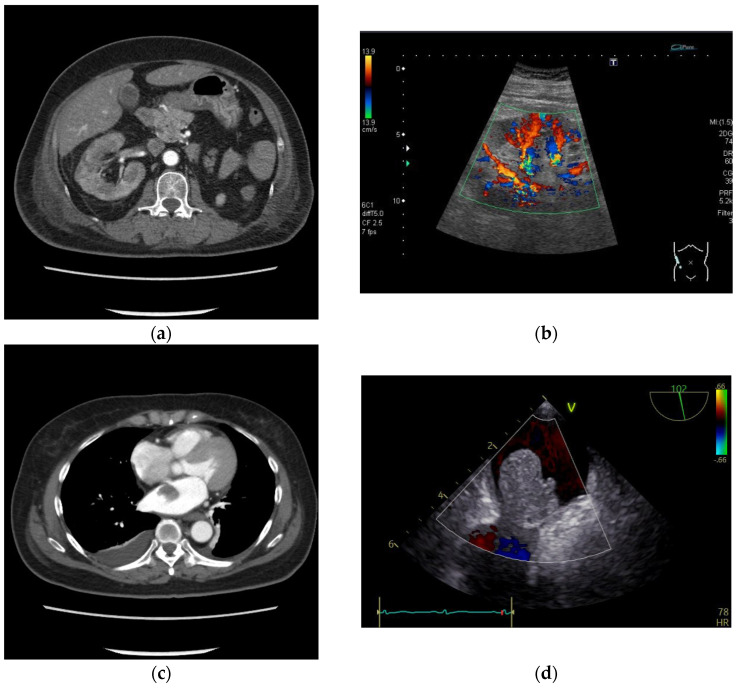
The patient’s enhanced abdominal computed tomography scan (a), renal ultrasonography (b), enhanced chest computed tomography scan (c), and transesophageal ultrasonography (d).
